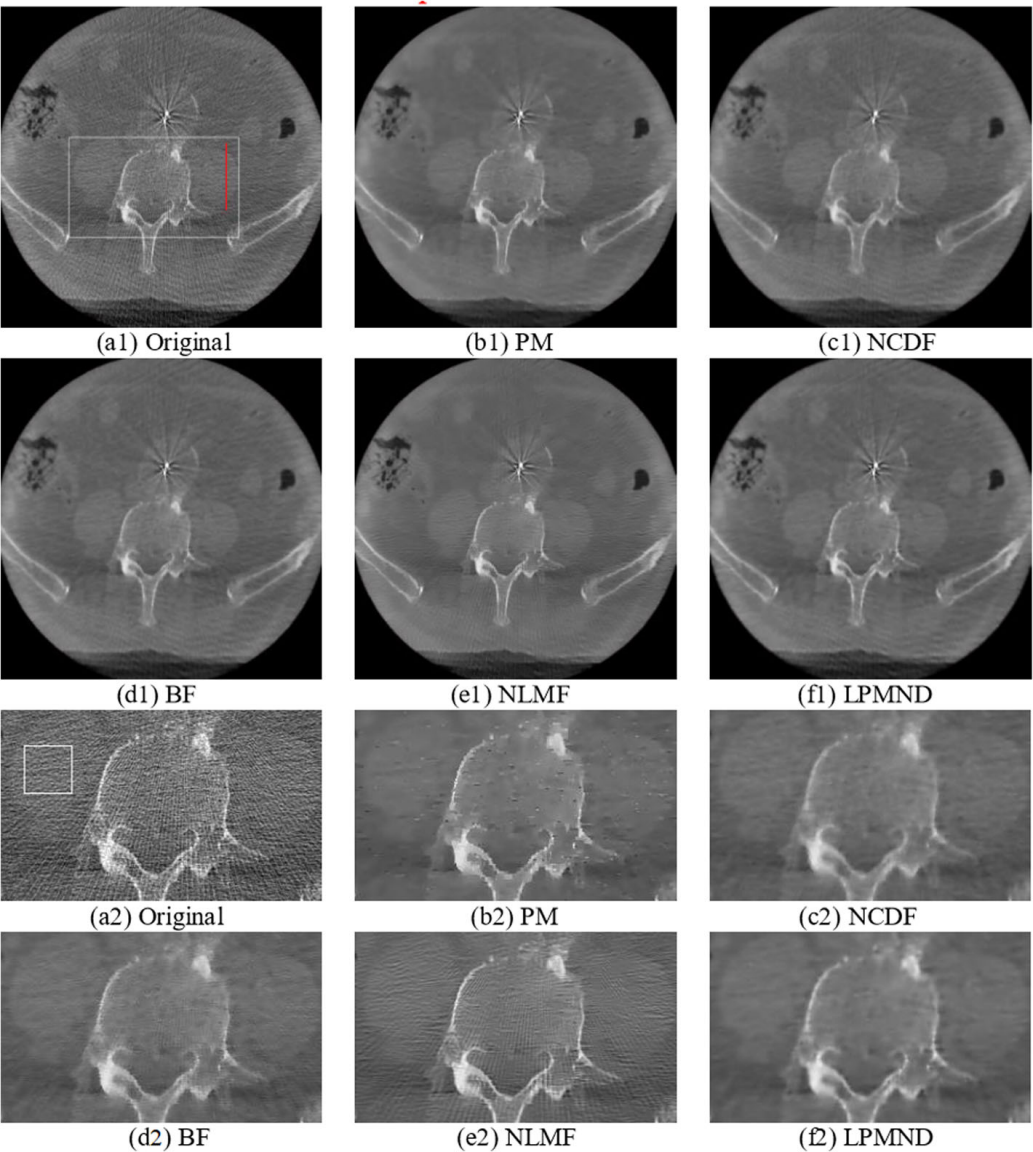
One slice of the CBCT data from one patient and its enlarged region which is indicatd by a white square in (a1). a1 the original image, b1-f1 filtered image by PM, NCDF, BF, NLMF and LPMND, respectively. a2-f2 Corresponding enlargements in (a1-f1). Display window: [− 1024 HU, 1976 HU]
